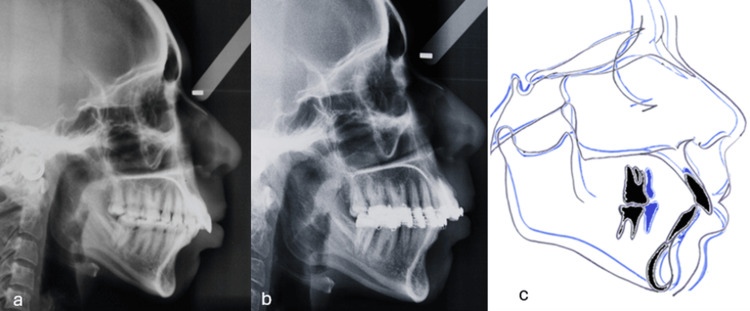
Lateral cephalometric radiographs. (a) Pre-treatment. (b) Presurgical after orthodontic decompensation. (c) Superimposition of pre-treatment (black) and presurgical (blue) tracings.
